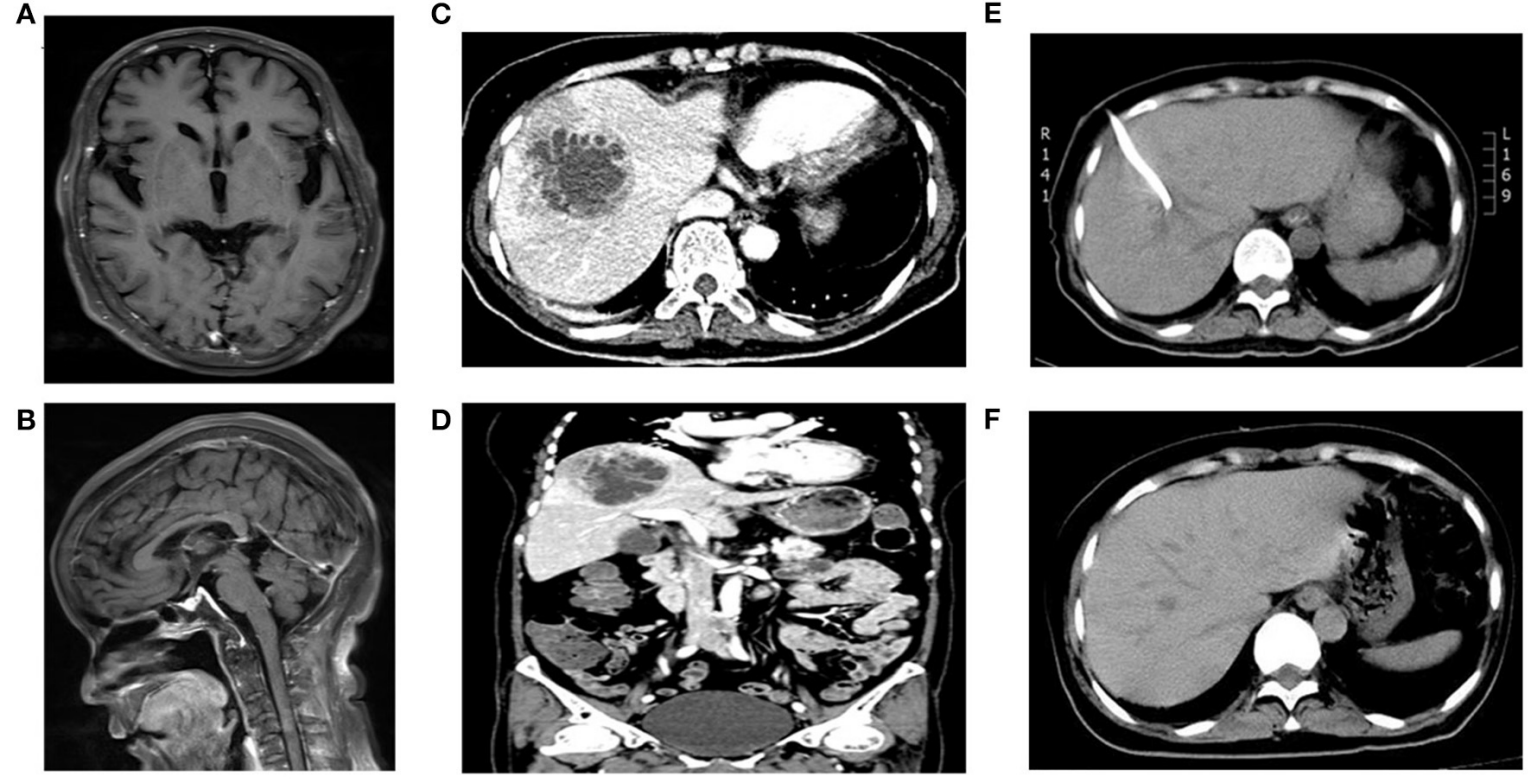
MRI or CT scan images of the patient. (A,B) indicated partial enhancement of pia mater in brain enhanced MRI; (C,D) revealed an area of abnormal attenuation measuring 67 mm × 62 mm in the right lobe of the liver, indicative of a single large multi-loculated abscess in abdominal enhanced CT; (E) displayed the abscess reduced to 51 mm × 37 mm 3 days after emergency CT-guided percutaneous drainage of the liver abscess in abdominal plain CT; (F) showed no lesions in liver in the reexamination of abdominal CT after full recovery.
